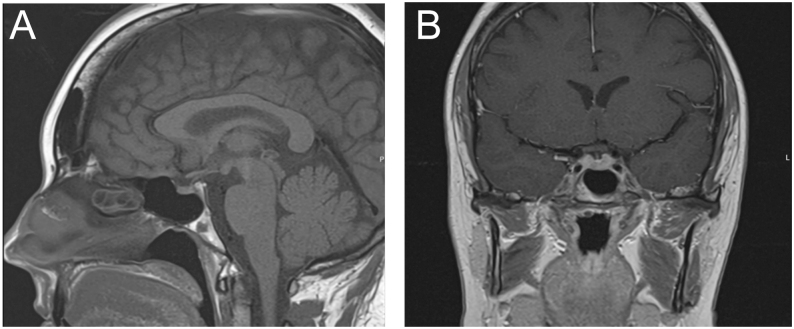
T1-weighted MRI of the pituitary demonstrating normal pituitary morphology in both sagittal (A) and coronal (B) views.
